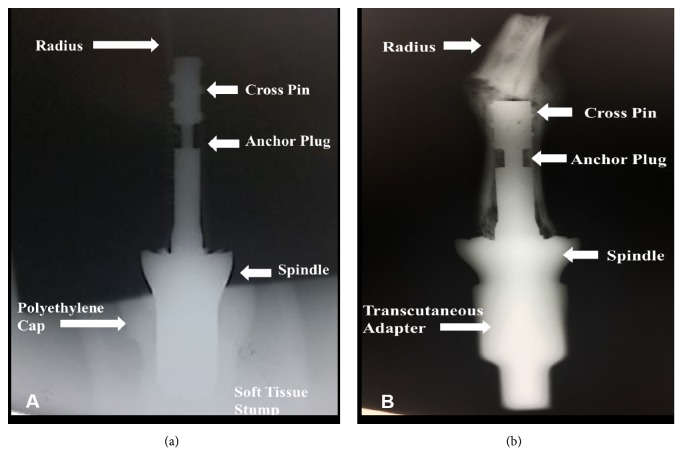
Radiographic images of the staged implant procedure. (a) Implant of spindle components with polyethylene cap and primary wound closure (b) Removal of polyethylene cap which is replaced with a tapered-fit transcutaneous adapter.
